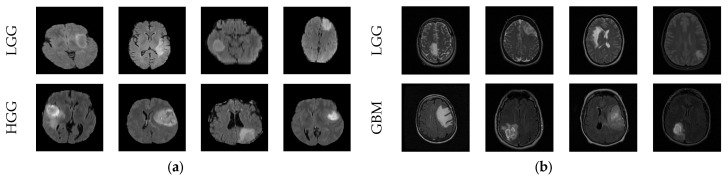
Sample test images of BRATS and TCIA. (a) FLAIR-modality MRI slices collected from BRATS, (b) T2-modality MRI slices collected from TCIA.
